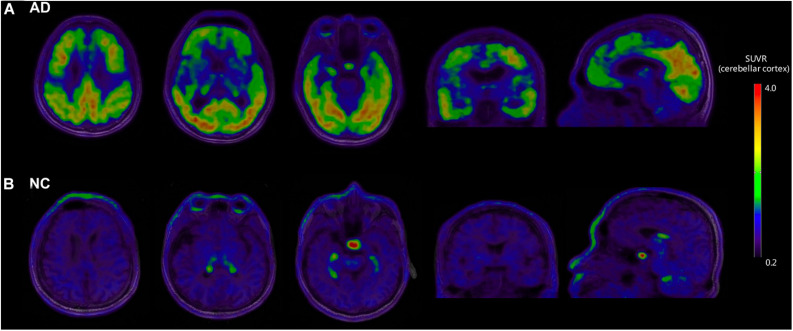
Representative examples of [^18^F]-APN-1607 PET and anatomical MRI superimposed images of AD and NC subjects. (A) [^18^F]-APN-1607 PET of an AD patient (male, 56 years old, MMSE 17, 11 years’ education experience, memory impairment complaint for 5 years with positive [^11^C]-PiB result). (B) [^18^F]-APN-1607 PET of a NC subject (Male, 61y). AD, Alzheimer’s disease; NC, normal control. The color stripe indicates the standard uptake value ratio with cerebellar cortex as the reference region.
